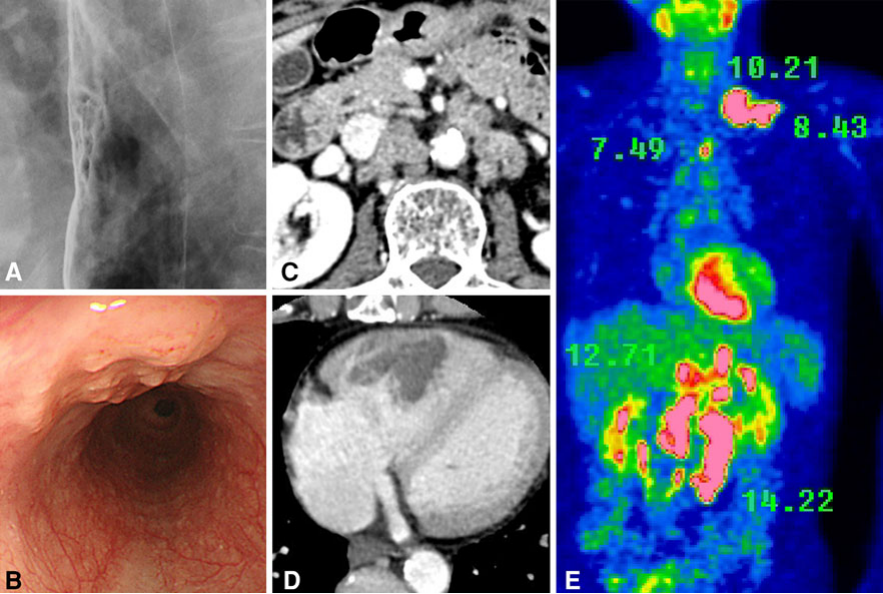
Esophageal squamous cell carcinoma was detected in the middle thoracic esophagus (a, b). Contrast-enhanced CT showed the multiple para-aortic lymph node metastases and cardiac right ventricle metastasis (c
